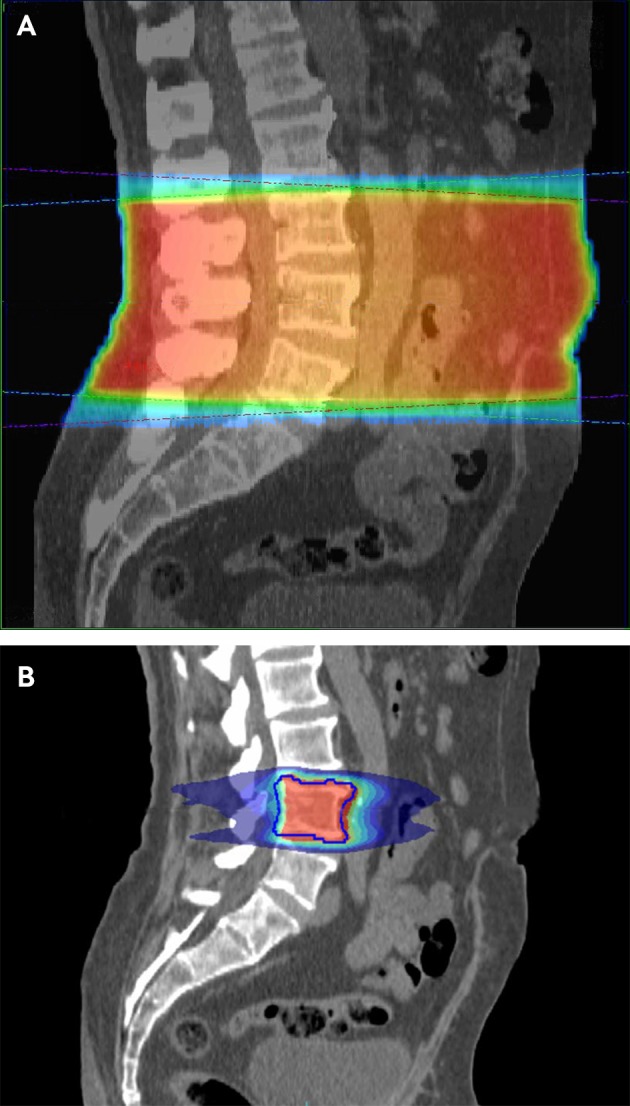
Computed tomograms showing the difference in radiotherapy dose distribution between simple, conventional palliative radiotherapy (A), and targeted stereotactic radiotherapy (B). The latter treatment plan allows a dose of roughly three times greater biological effectiveness to the target with significantly lower dose to surrounding tissue
